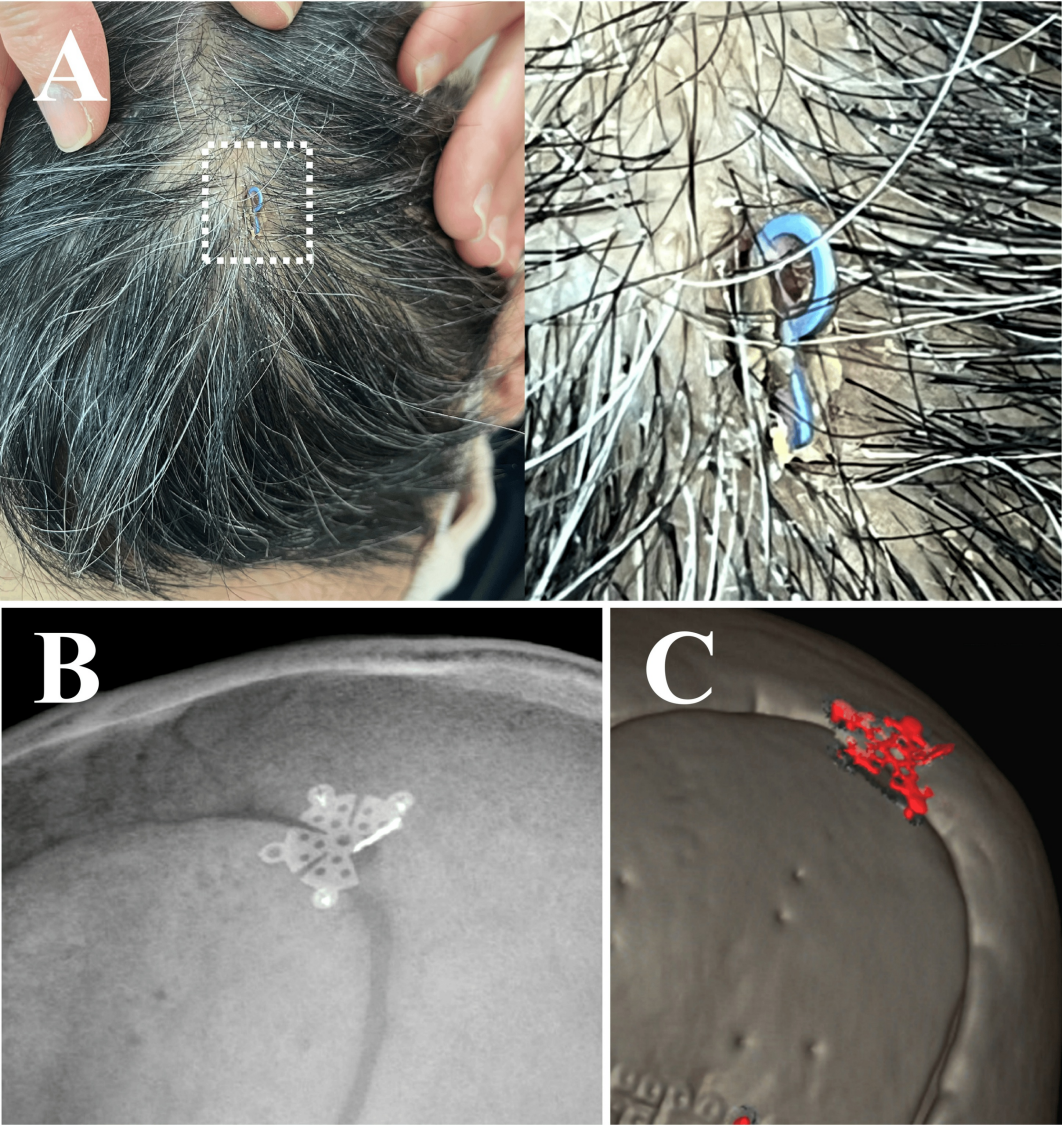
Titanium mini-plate protruding through the scalp A: A light blue metal fragment is seen protruding through the left parietal scalp, showing a screw hole contaminated with sebum. B, C: Plain radiography and three-dimensional computed tomography showed that a pinwheel-shaped, mini-titanium plate covering the burr hole had been fixed using only three screw holes. The unfixed wing was bent upward.
